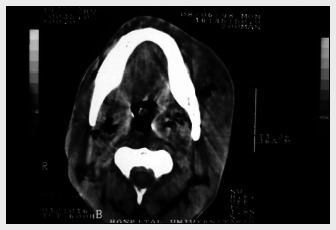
Cervical computed tomography scan showing gas in lateropharyngeal space, and also parapharyngeal and retropharyngeal bilateral abscess.
